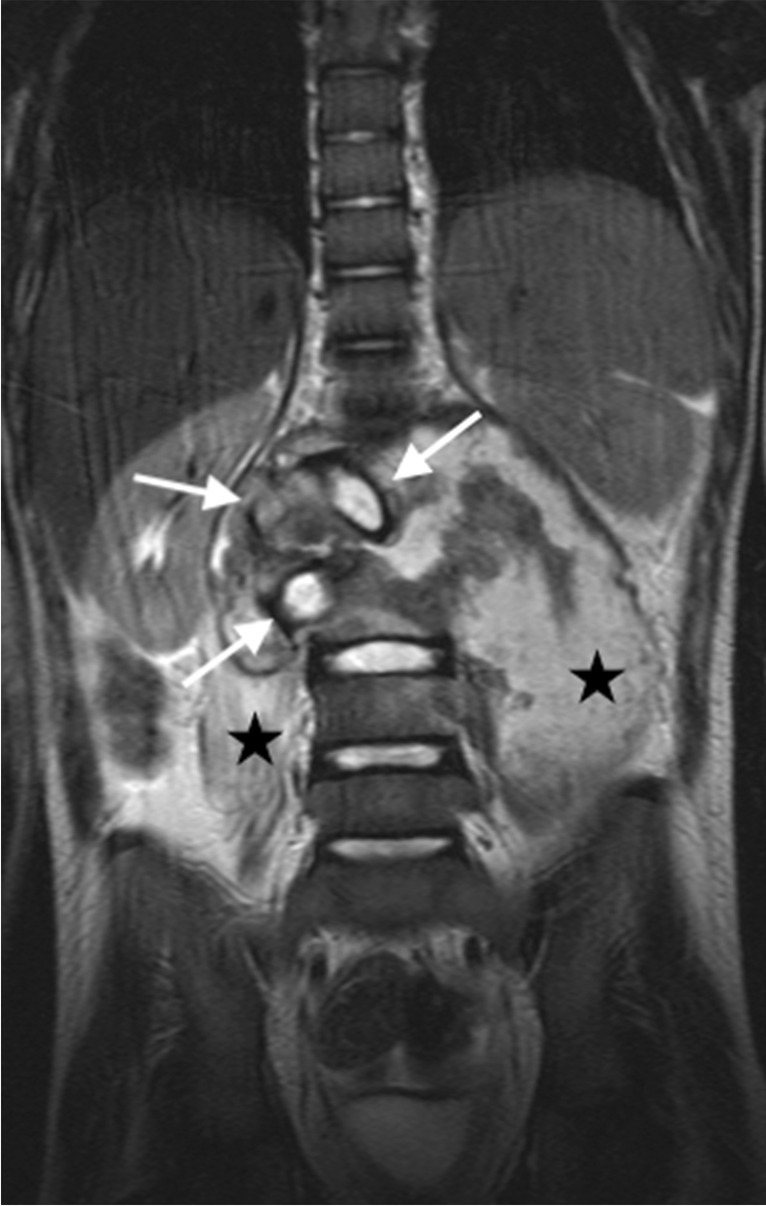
A coronal T2 magnetic resonance image in an 8-year-old girl with tuberculous spondylitis shows expanded discs with normal signal (*arrows*) subsumed within a large paraspinal collection that extends into both psoas muscles (*asterisks*)
